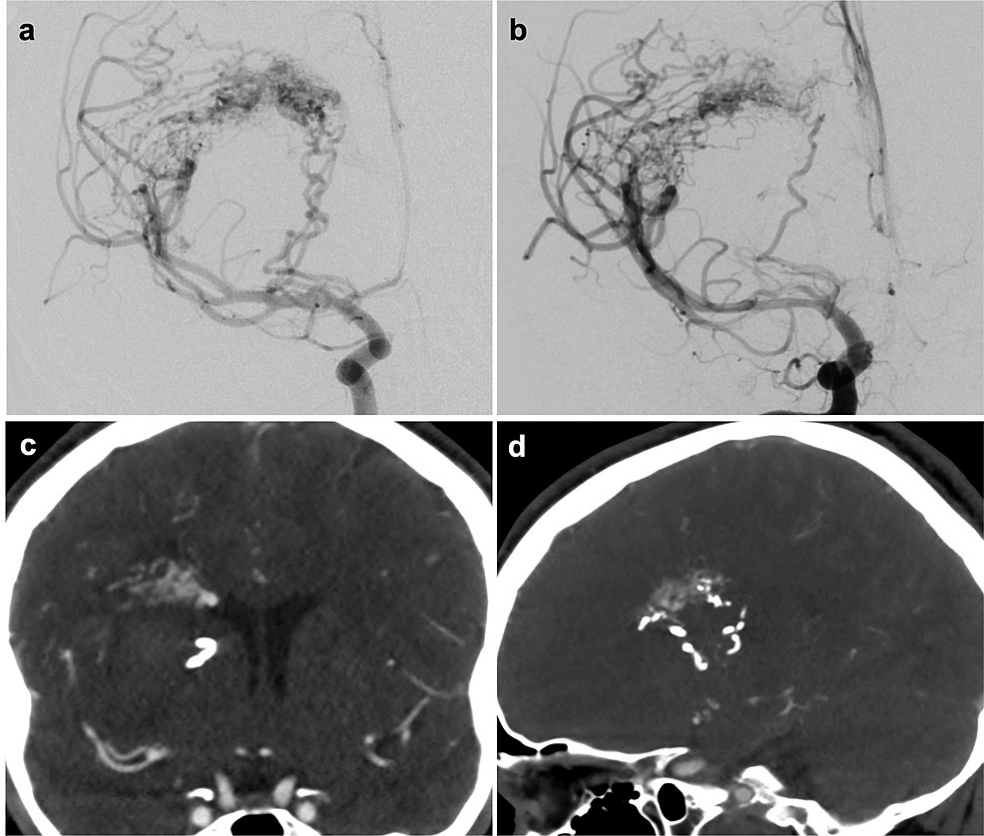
Angiogram and CTA post-embolization. Right ICA angiogram demonstrating (A) pre- and (B) post-embolization of two lenticulostriate artery feeders. Coronal (C) and sagittal (D) CTA demonstrating embolized AVM arterial feeders. CTA, computed tomography angiography; ICA, internal carotid artery; AVM, arteriovenous malformation.
